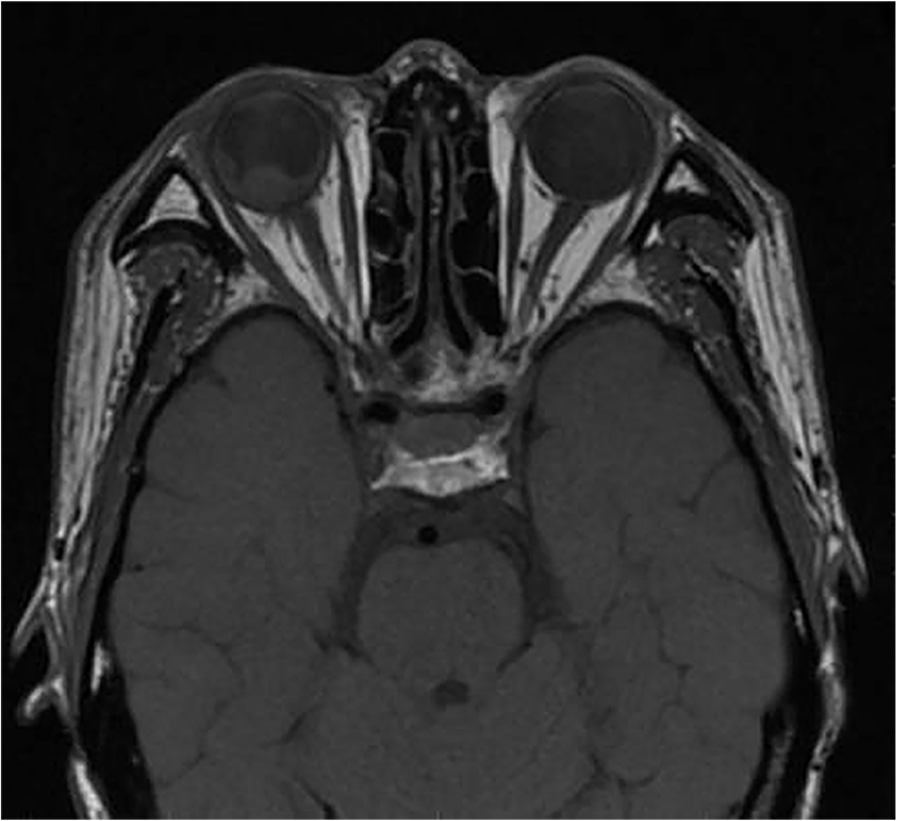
Transverse T1-weighted images revealed the presence of a right intraorbital fusiform mass with hyperintense on STIR sequence. The posterior wall was significantly thickened. The right optic nerve and peripheral fat gap were well-defined, and there was no obvious change in the extraocular muscle
